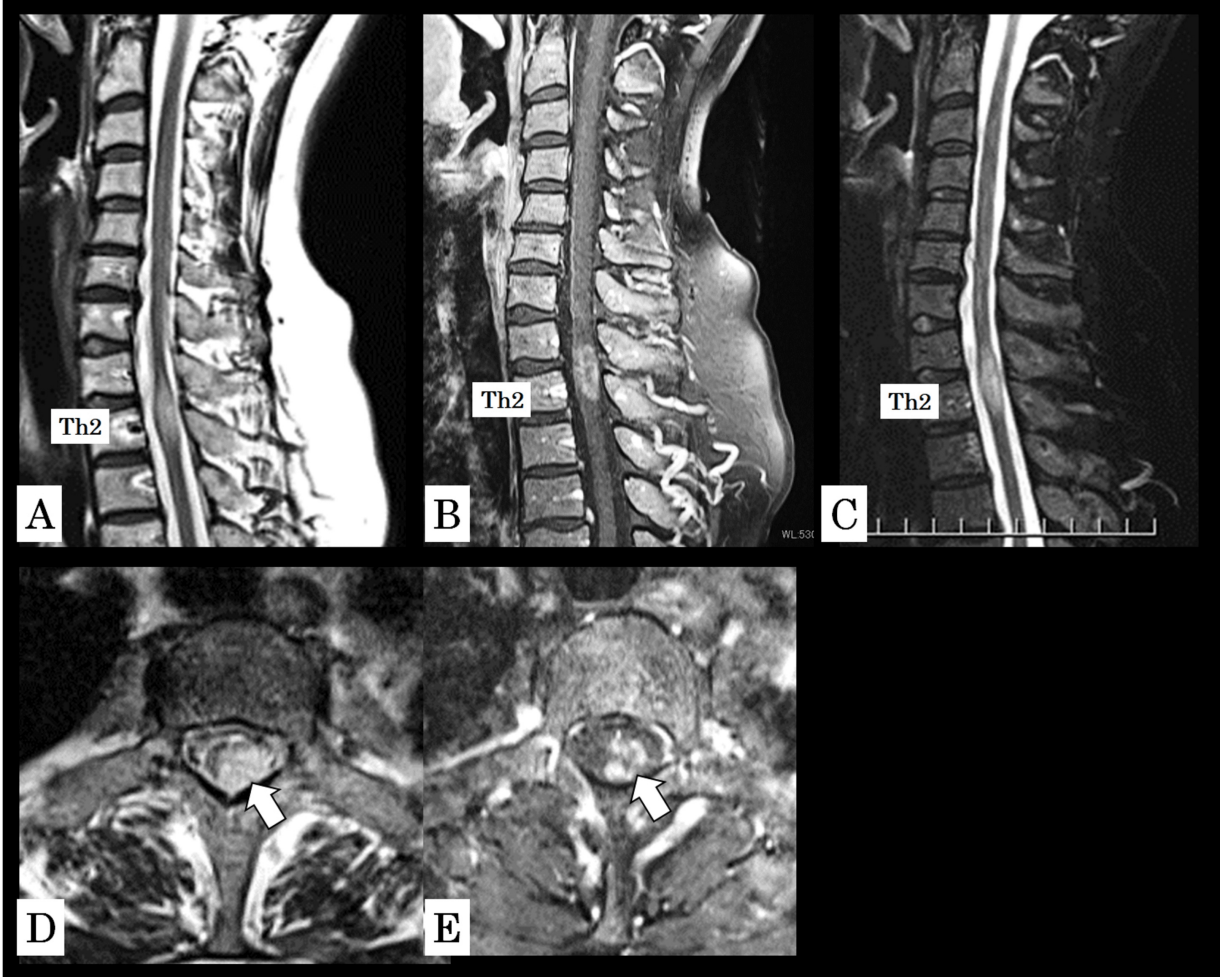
Cervical spine MRI findings. (A) T2-weighted sagittal image showing an intramedullary hyperintense lesion at Th1-2. (B) Gadolinium-enhanced sagittal image demonstrating a relatively well-demarcated intramedullary mass with mildly heterogeneous enhancement. (C) Fat-suppressed sagittal image in which the intramedullary high signal appears more conspicuous, with accentuated contrast against the surrounding spinal cord. (D) Axial T2-weighted image and (E) axial gadolinium-enhanced image showing the lesion at the corresponding level (arrows indicate the lesion).
